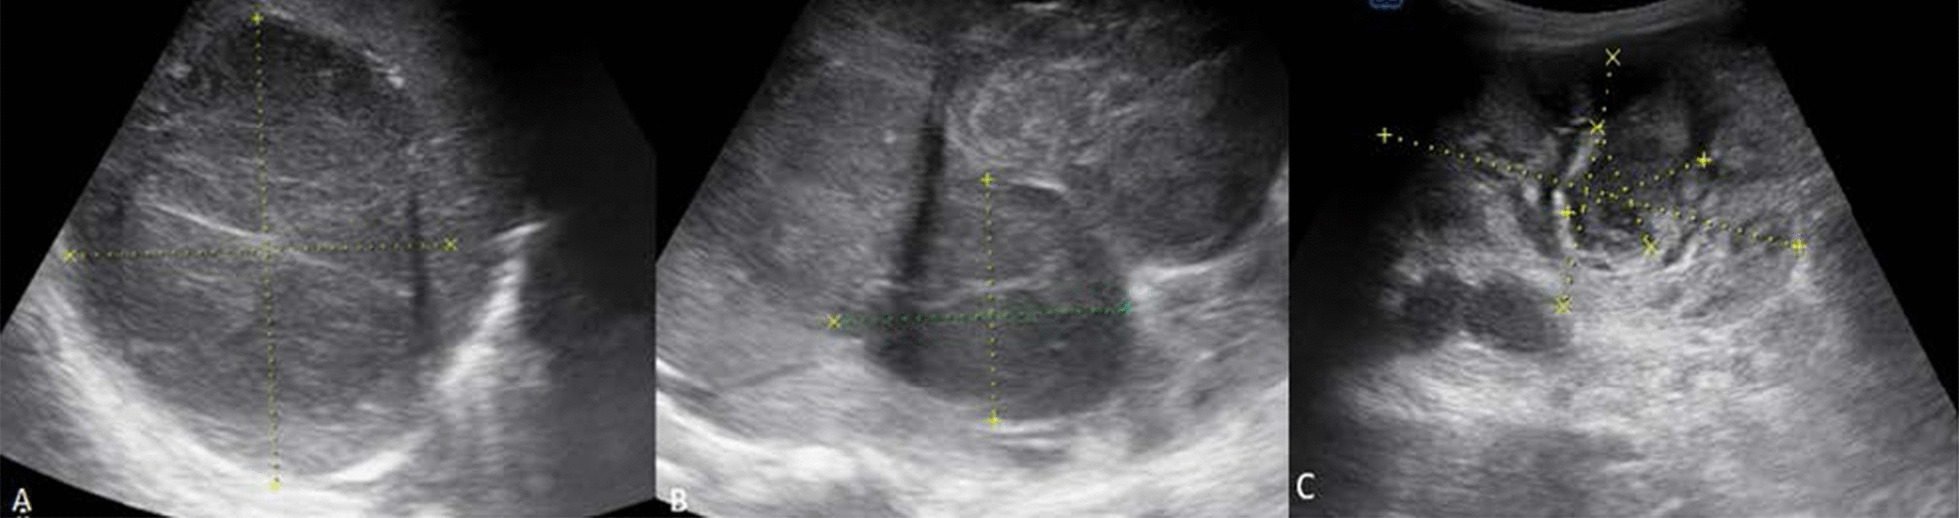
Ultrasound scan of the abdomen. A Heterogeneously hypoechoic solid mass in the right lobe of the liver in segments V, VI, VII, VIII; B heterogeneous solid mass in the middle to inferior pole of the right kidney; C echo complex mass predominantly solid in the left kidney
